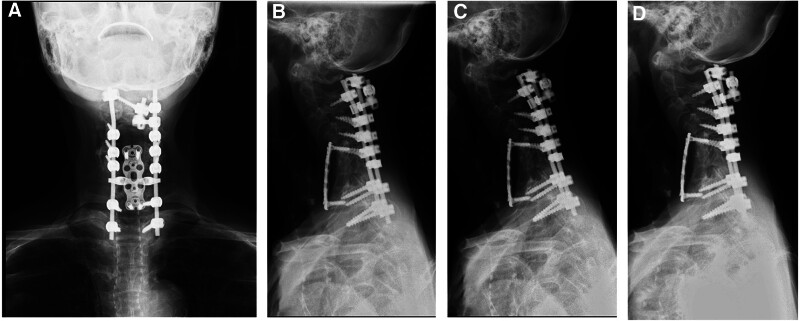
Cervical spine X-ray of the final observation. (A) The anteroposterior view; (B) the lateral view of the neutral posture; (C) flexion; (D) extension.
